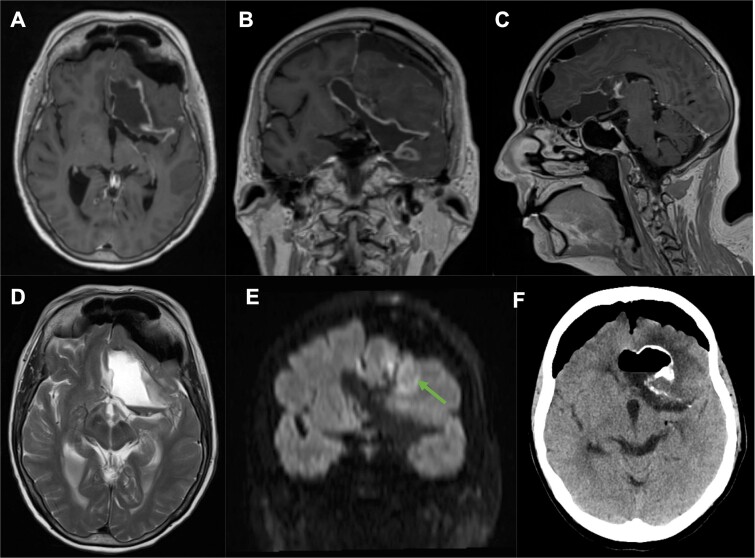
Post-operative MR T1 post gadolinium [axial (A), coronal (B), sagittal (C)], axial T2 (D), coronal DWI B1000 (E) and axial CT (F) confirmed complete evacuation of the dermoid cyst contents with the capsule preserved *in situ*.
